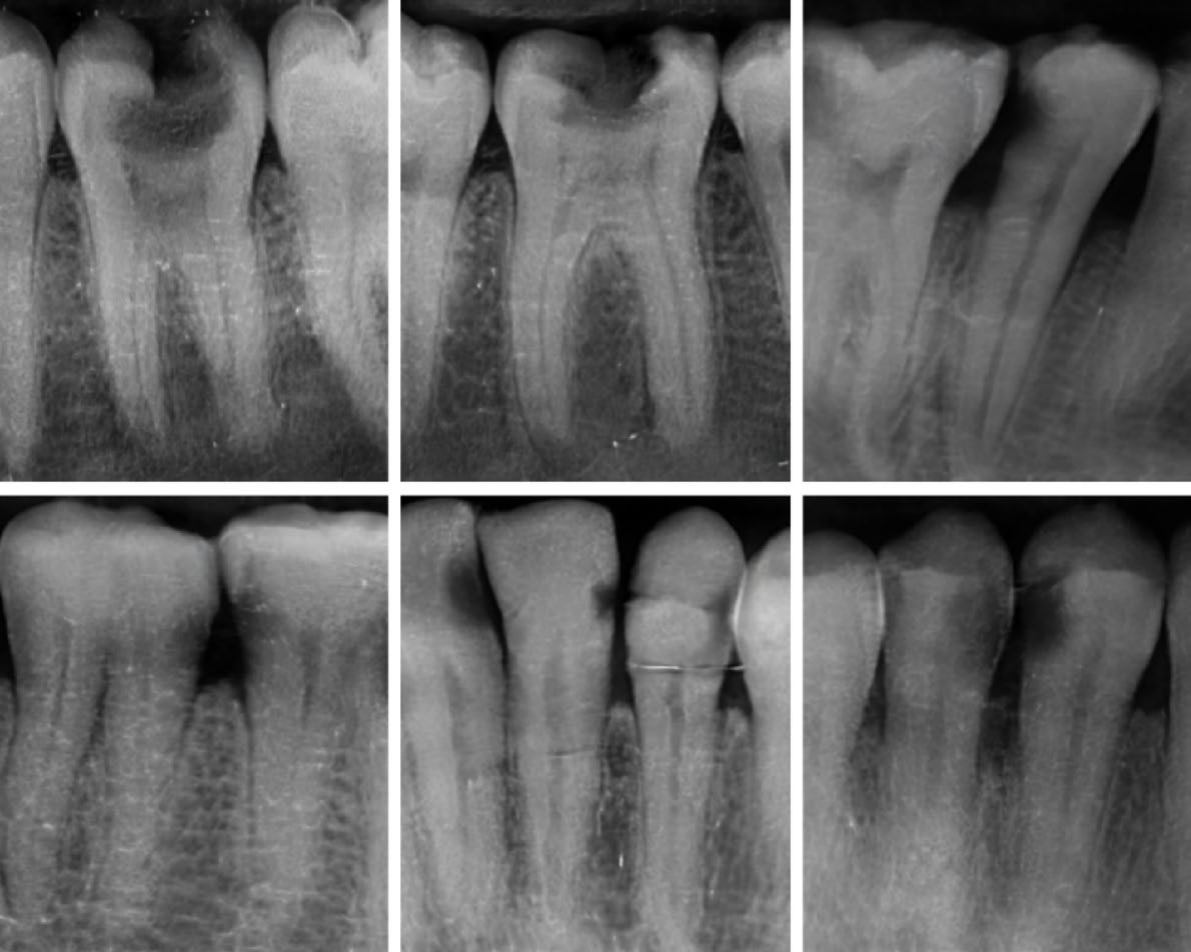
Representative synthetic intraoral periapical radiographs generated using the StyleGAN2-ADA frame work.
